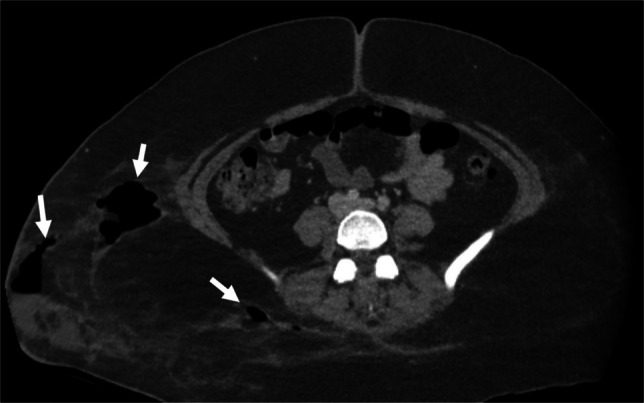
Necrotizing fasciitis. A 15-year-old girl with a history of a motor vehicle collision and abrasion to the right flank 1 month prior presented to the emergency department with concerns for a wound infection. Axial CT with contrast shows multiple air-filled collections within the soft tissues of the right flank (*white arrows*). The most lateral collection has an air-fluid level
